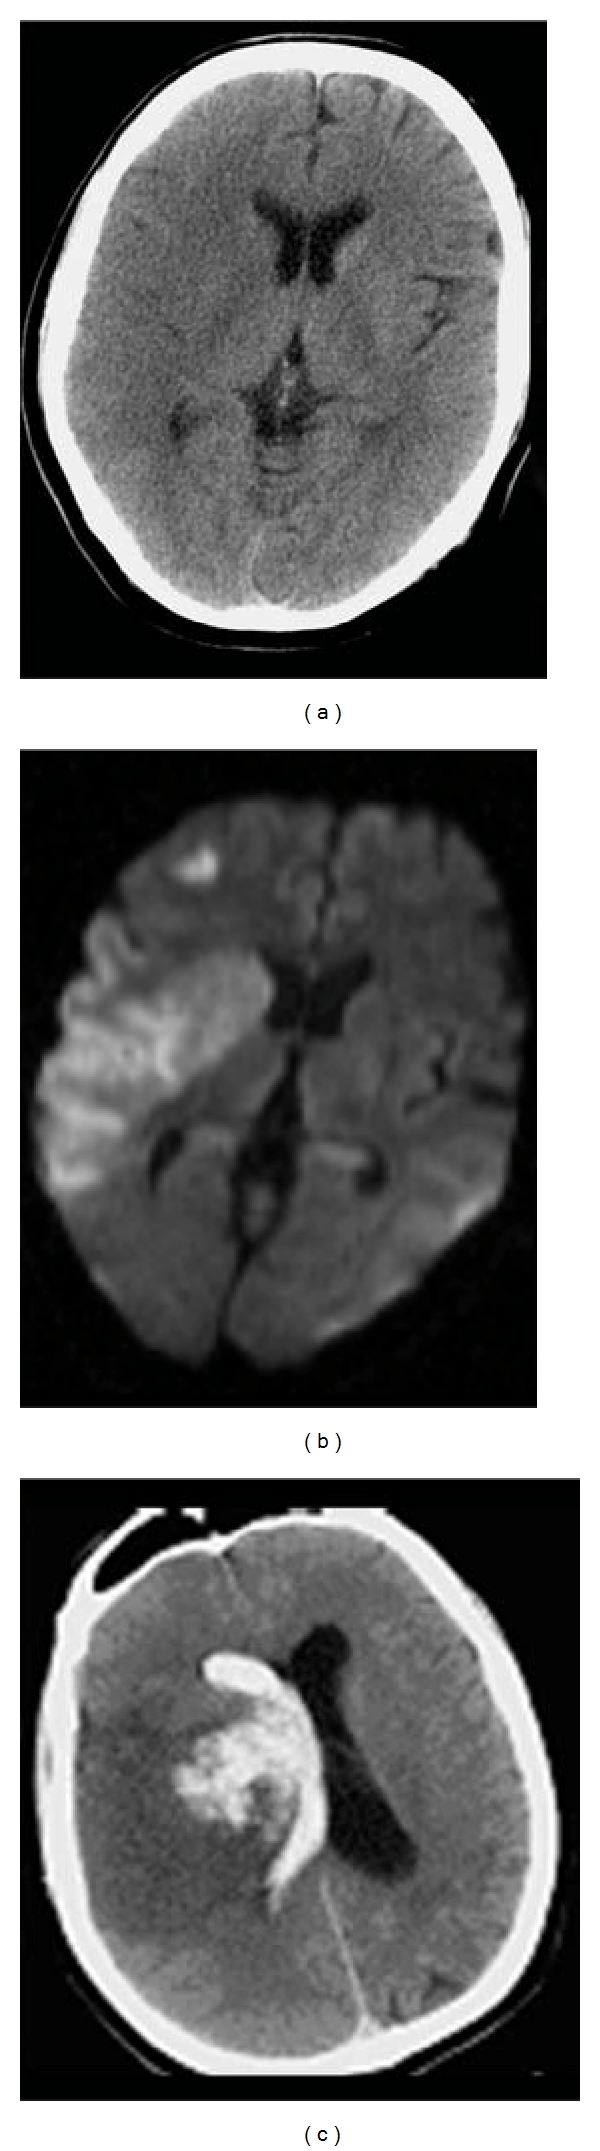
55-year-old man presented with dense left hemiparesis one week after right carotid stenting. (a) Initial axial unenhanced CT shows a large area of low attenuation in the right middle cerebral artery territory. (b) axial DW-MR image 2 hours later confirms a large infarct in the striatocapsular region, including the right caudate head. (c) Axial unenhanced CT 12 hours later shows a large parenchyma hemorrhage within the wedge-shaped area of infarction, consistent with hemorrhagic transformation. The case demonstrates two features typical of hemorrhagic infarct: the involvement of the caudate head and the distribution of the oedema surrounding the stroke lesion following the affected arterial territory. Stage IIH d2 [1].
